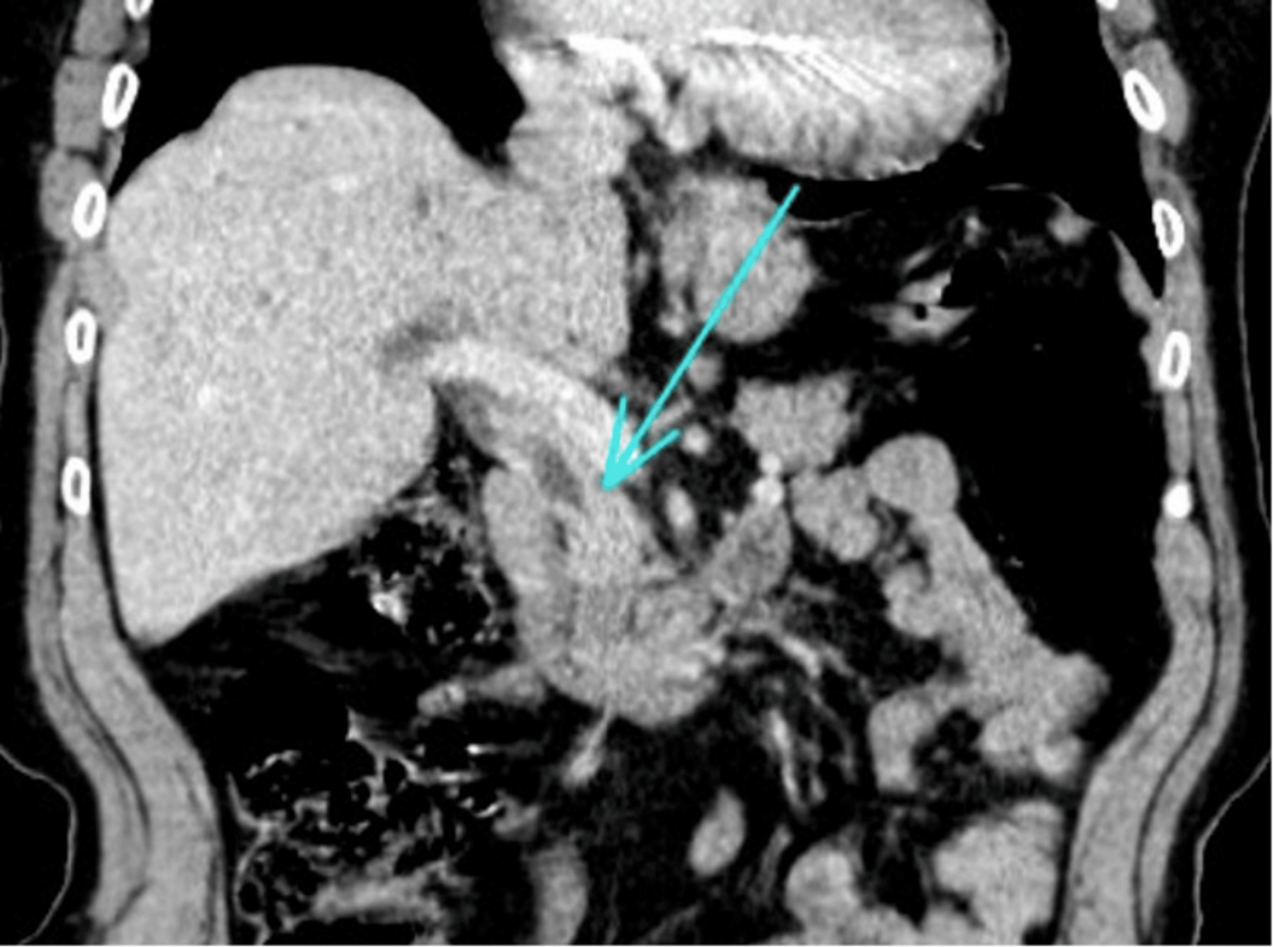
CT showing biliary dilatation with common bile duct thickening and abrupt transition and stricturing at the distal end.
